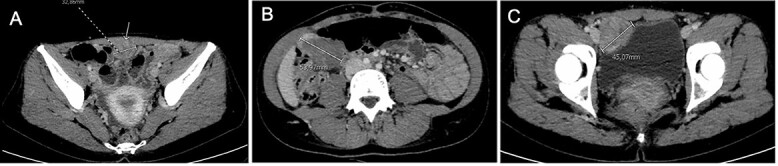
Computed tomography at diagnosis showing the three tissular lesions adherent to the anterior abdominal wall. (A) Tumor in contact with infra-umbilical linea alba. (B) Lesion located at the right hypochondria. (C) Tumor situated in the right iliac fossa near the bladder.
